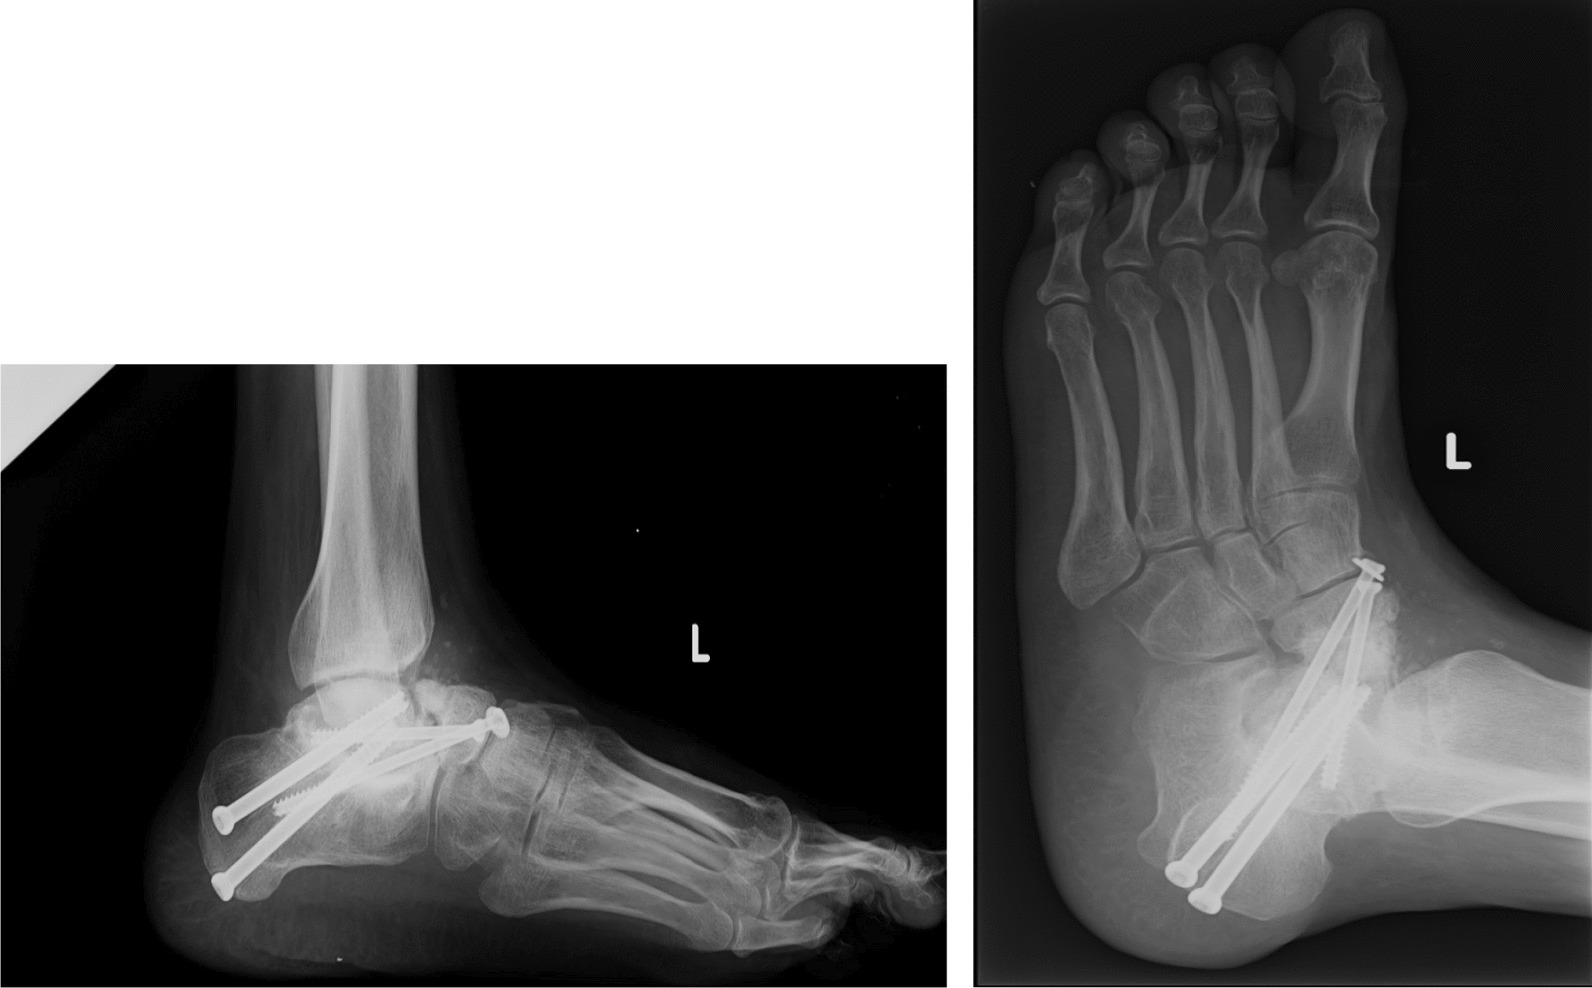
3 months x-ray after therapy
